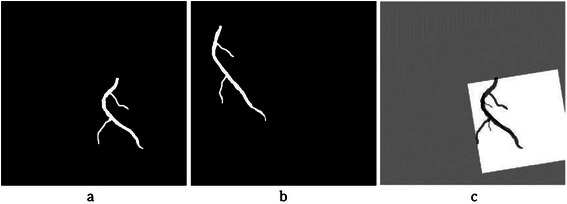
The results of registration without control point for two selected images. (a) Image *A* from CTA as the fixed image. (b) Image B from angiogram as the moving image. (c) The result of applying affine transformation without control point.
